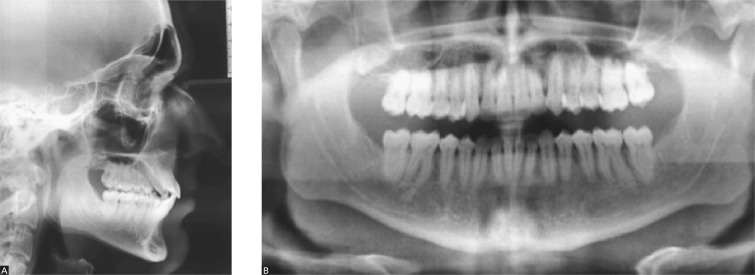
Radiographs after 5 years in retention. A) Cephalometric radiograph; B)
